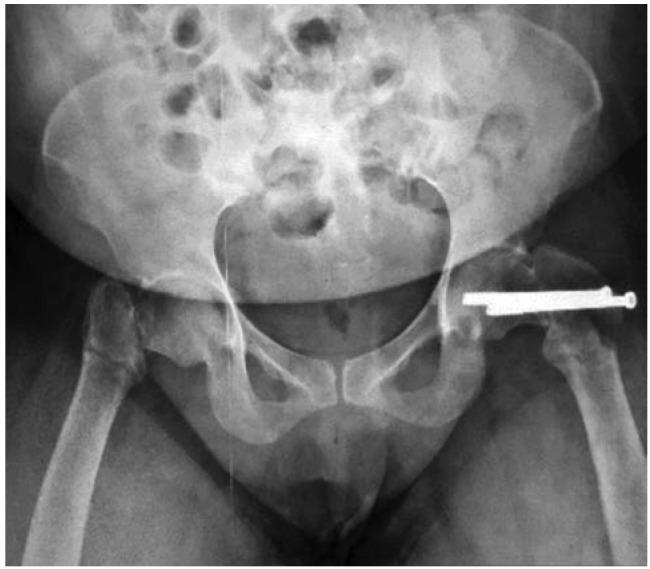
Pelvis AP radiograph at admission showing right sided transcervical neck of femur fracture with left intertrochanteric fracture. Two screws intact in the left neck of femur from previous fixation.
